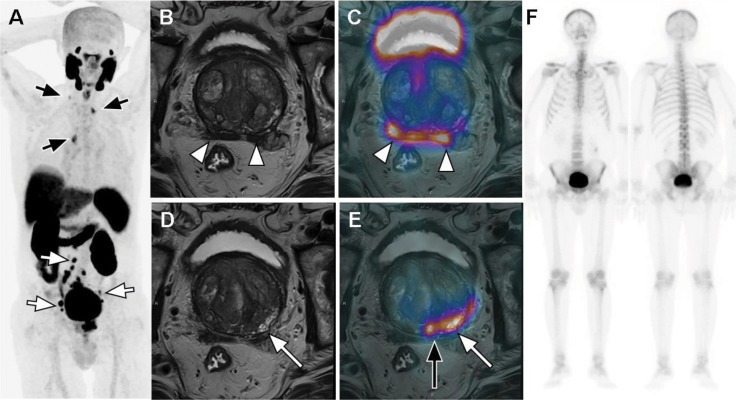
PSMA PET/MRI for initial staging of a 77-year-old high-risk PCa patient with a Gleason score of 7 (3+4) and a PSA of 44.0 ng/dL. The staging would be T3bN1M0 if the patient was assessed with conventional imaging, namely, mpMRI (B and D) and BS (F). PSMA PET (coronal maximum intensity projection (MIP)inf A and fused PET/MR in C and E) clearly depicts more extensive pelvic (white arrows in A), extrapelvic thoracic and right inferior cervical nodal involvement (black arrows in A), upstaging the patient to T3bN1M1a. The incremental value of multimodal assessment is also seen in terms of the patient's T status. There is concordance between PSMA PET and MRI regarding bilateral seminal vesicle involvement encompassing the whole right seminal vesicle and the medial portion of the left seminal vesicle (white arrowheads in B and C), whereas PSMA PET/MRI revealed a more extensive (black long arrow in E) primary lesion in the left and median peripheral zones of base and midgland than mpMRI (white long arrows in D and E).
